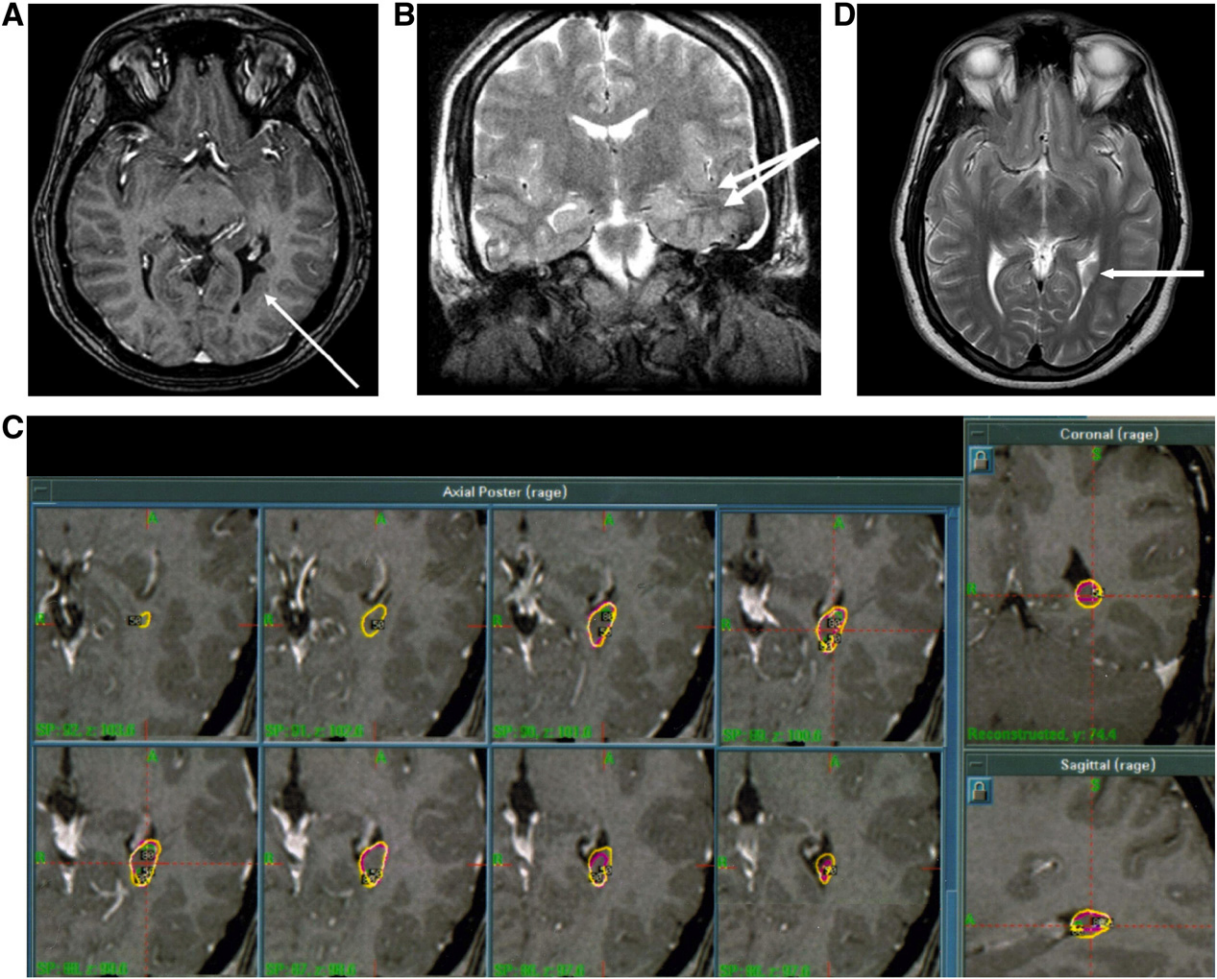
A: T1 MRI demonstrating PVH in the left trigone. B: T2 Coronal MRI status-post implantation of intracranial electrodes. C: Dose plan for Case 1. D: Axial T2 MRI showing left trigone PVH.
